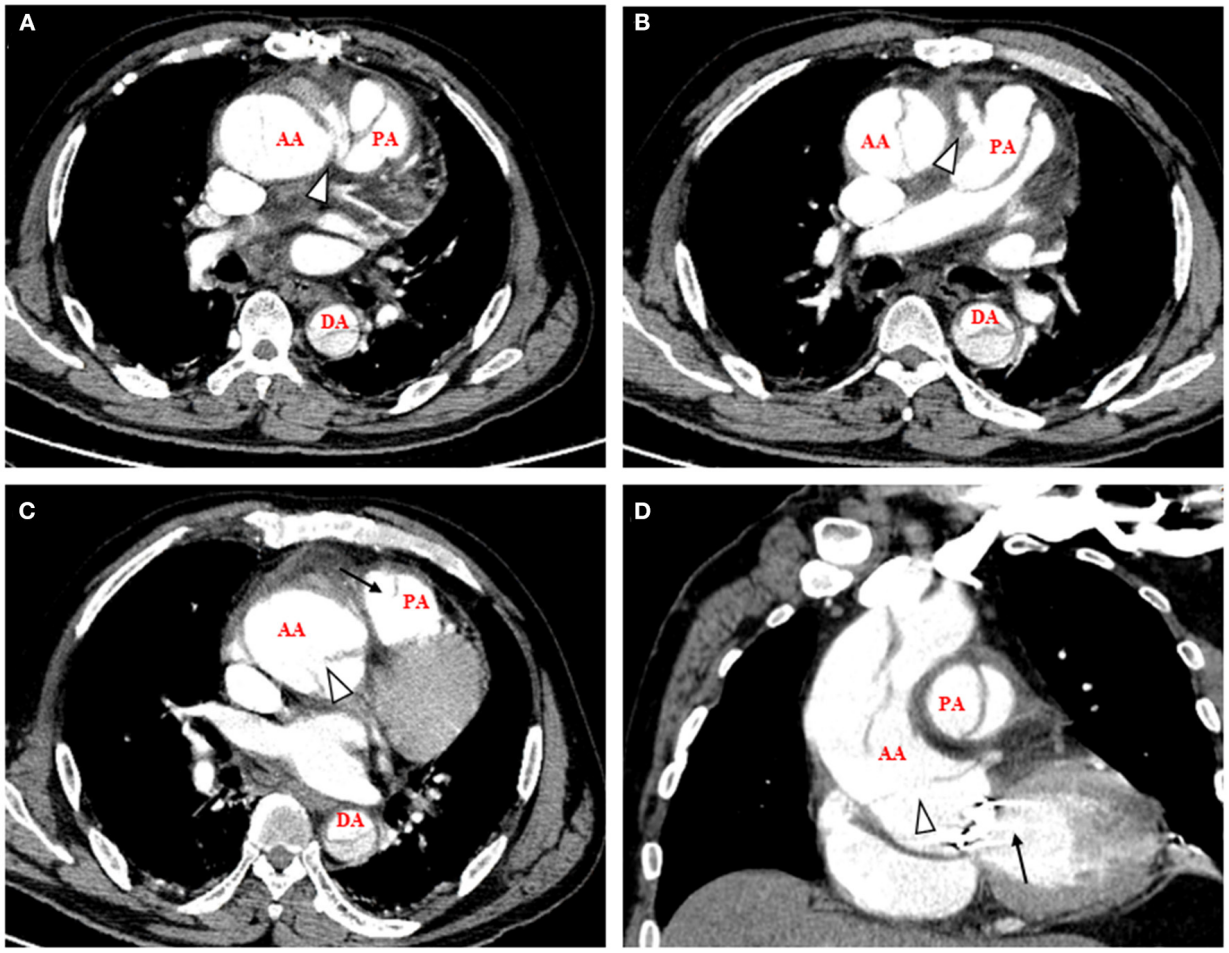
CT angiography on admission showed a simultaneous ascending aortic dissection and pulmonary artery dissection combined by aortopulmonary fistula. (A) Axial view, arrowhead showed the aortopulmonary fistula near the aorta root. (B) Axial view, arrowhead showed the aortopulmonary fistula near the PA. (C) Axial view, arrowhead showed the intimal flap of AA and arrow showed the intimal flap of PA. (D) Sagittal view, arrowhead showed the intimal flap of AA that was in the aorta root and arrow showed the artificial aortic valve. AA, ascending aorta; PA, pulmonary artery; DA, descending aorta.
